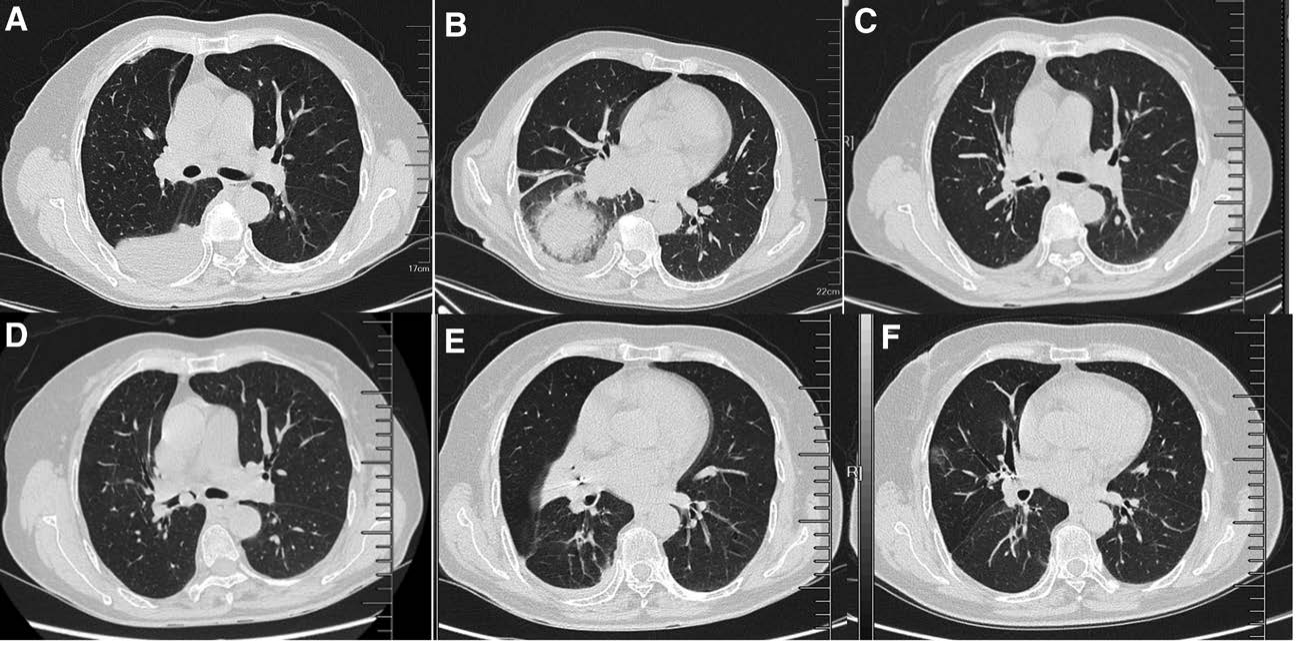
Chest CT of the patient. (A, B) CT: the presence of a right pulmonary mass, right lower lobar bronchus obstruction, and right pleural effusion. (C) CT: a new lesion appeared in the right intermediate bronchus. (D) CT: The lesion reobstruction in the right intermediate bronchus. (E) CT: ^125^I seeds were implanted in the lesion of the right middle lobe and 12R. (F) CT: lesion reduction and right middle lobe recruitment. CT = computed tomography.
